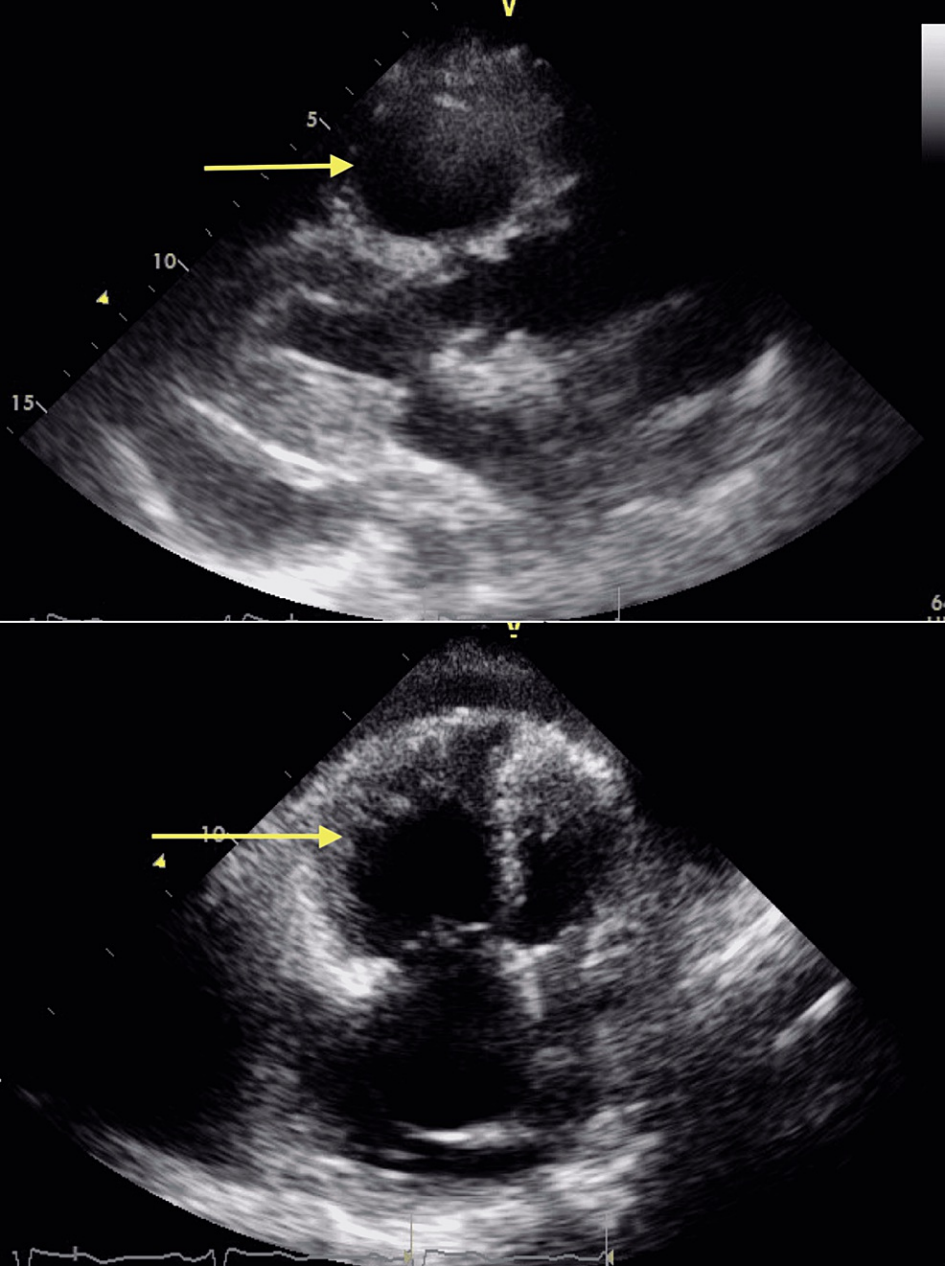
Echocardiogram showing moderate right ventricular dilation (arrows) with leftward diastolic displacement of the interventricular septum
